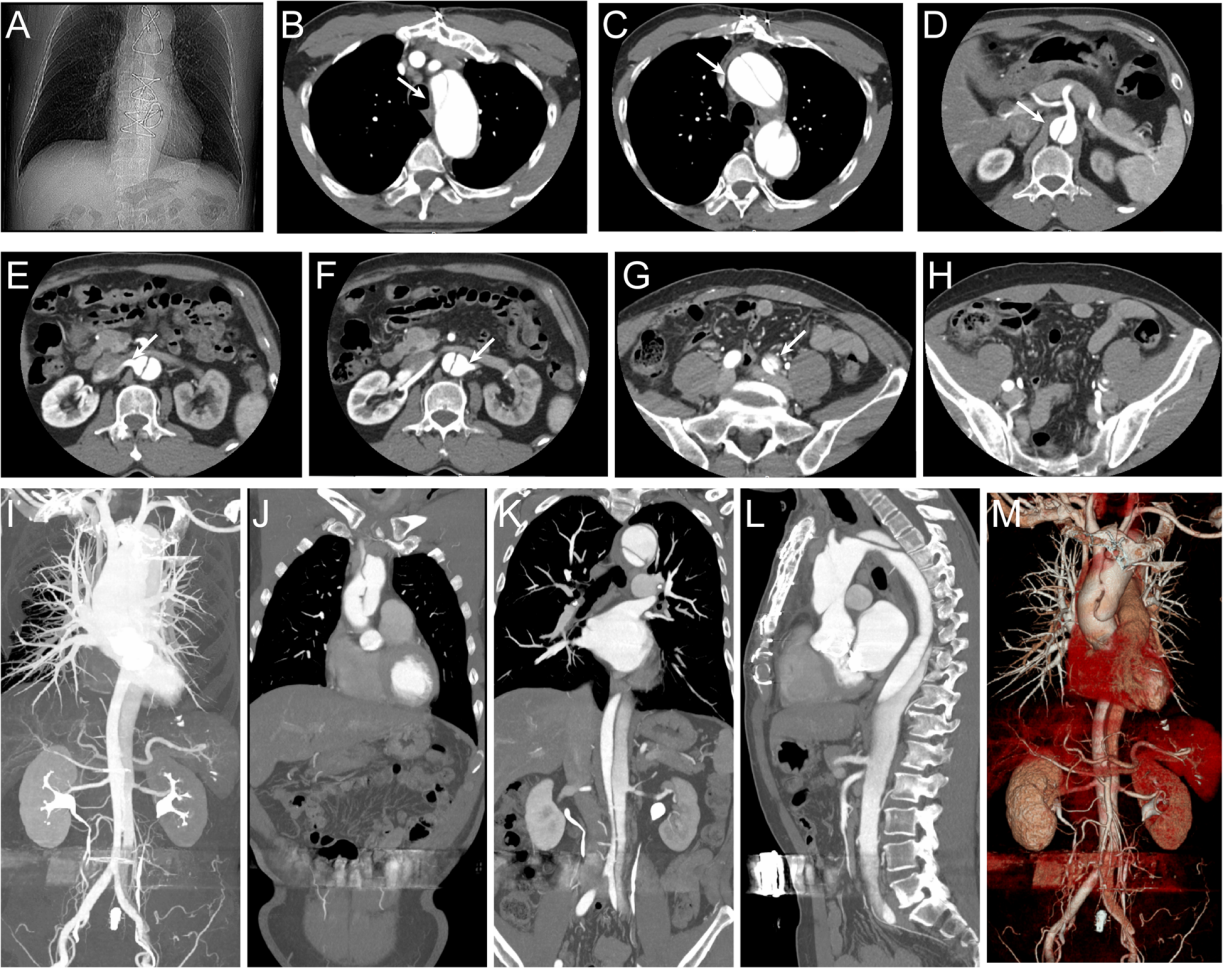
The iatrogenic ascending aorta dissection was identified by computed tomographic angiography (CTA) scan at the sixth month fellow up after aortic valve replacement (the arrow). (a-c) The site of dissection was about 4 cm above coronary ostium extending distally to iliac artery; (d-g) Blood supplying of superior mesenteric artery, right renal artery was from true lumen; (h-m) Blood supplying of left renal artery was from false lumen, and blood supplying of celiac artery was from true and false lumen
